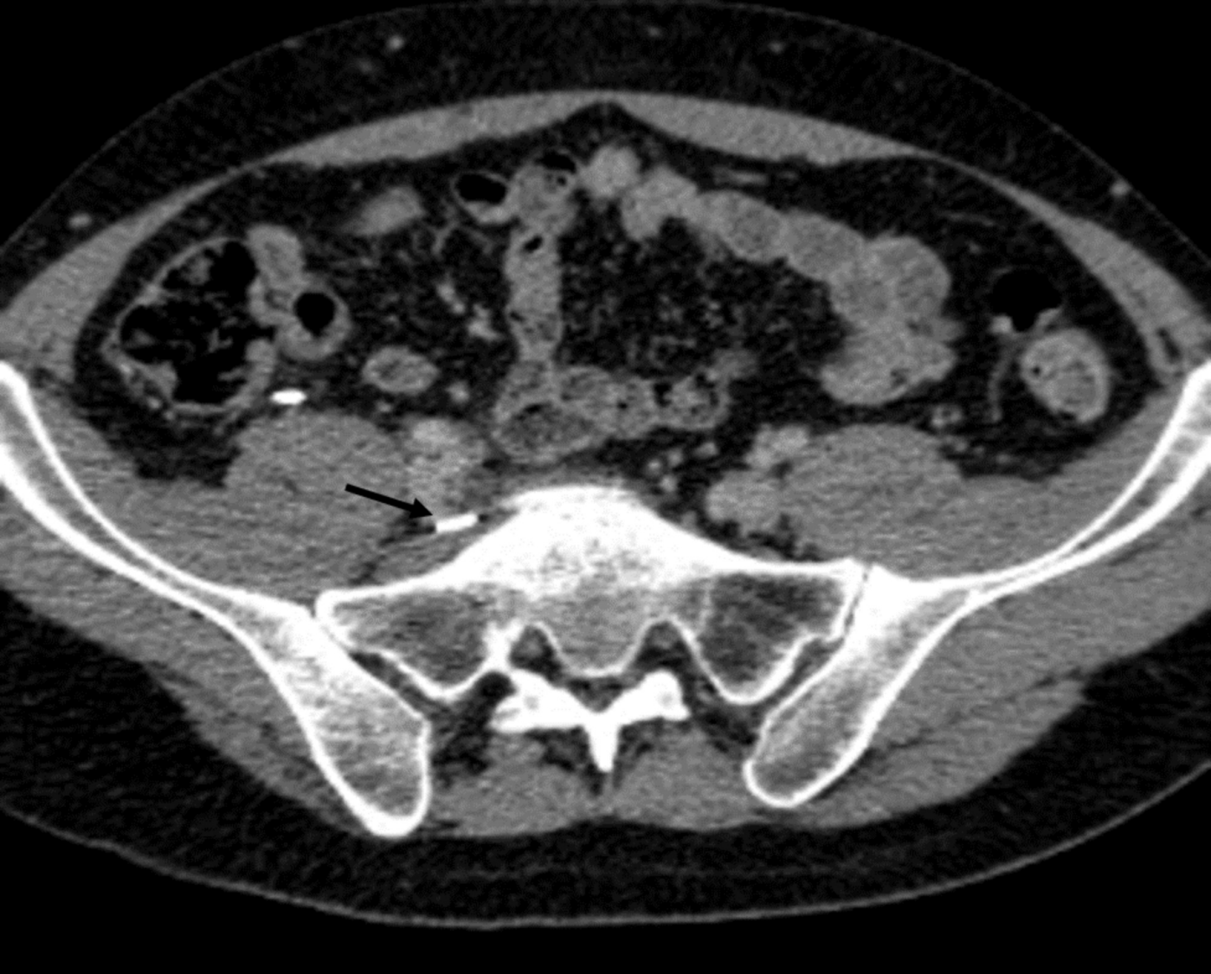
Post-operative CT Axial computed tomography (CT) demonstrating metallic clip anterior to the mass (black arrow)
